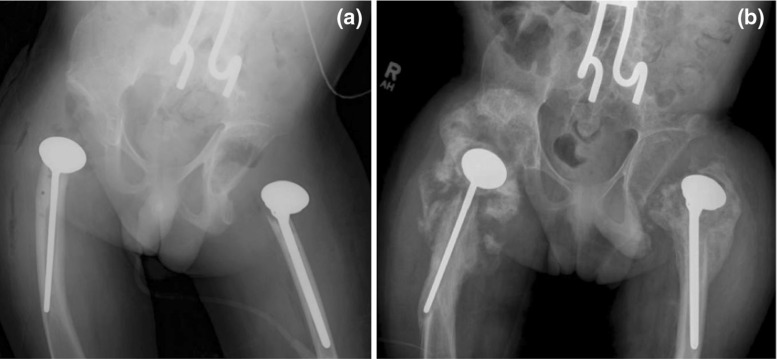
a Immediate post-operative radiograph of a child who underwent bilateral PFIA. b 25-month post-operative radiograph showing heterotopic ossification of the hips; however, the HO was not clinically significant and the child has yet to require revision surgery
